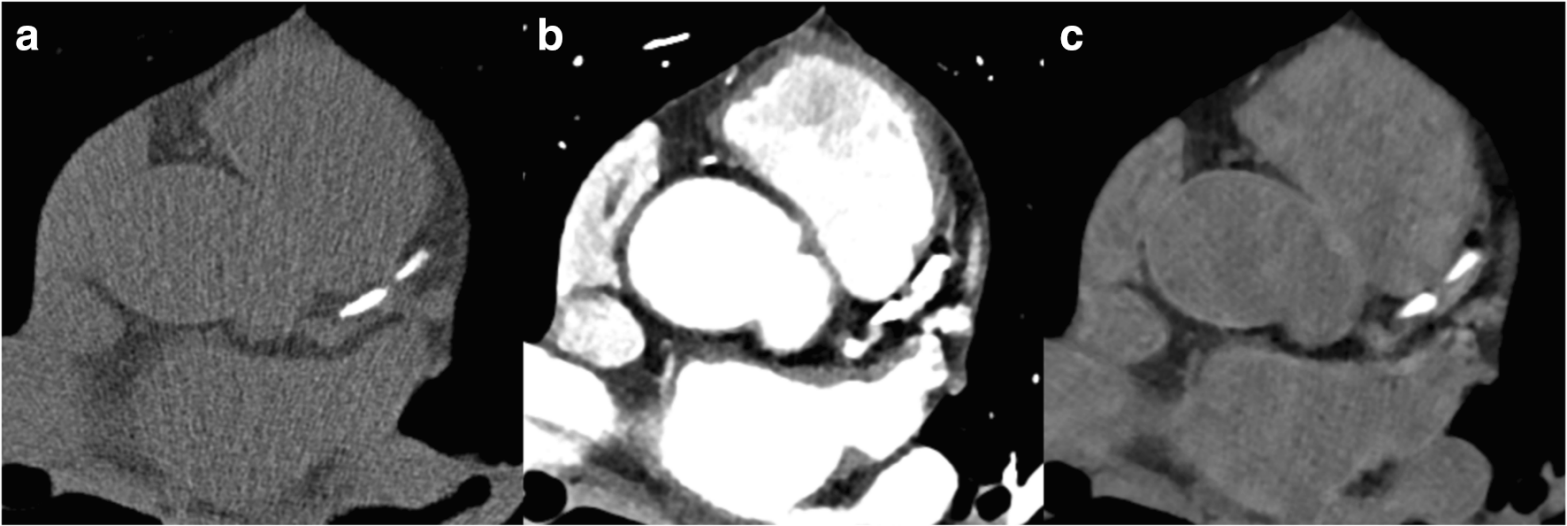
True non-contrast image from a native scan (a), contrast-enhanced image (b), and virtual non-contrast image (c) from a coronary computed tomography angiography (coronary CTA)
